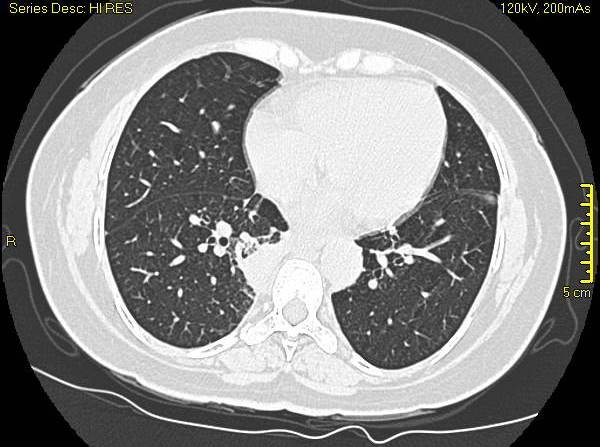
High resolution CT scan of the chest showing the lung nodules.
